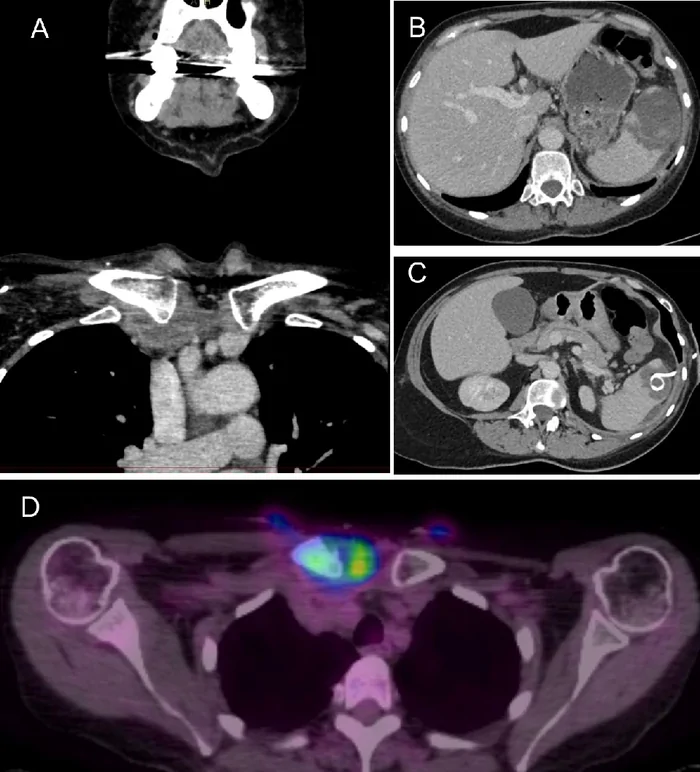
(A) Coronal reformation of a contrast-enhanced computed tomography (CT) scan shows thickening and hyperenhancement of the synovium of the right sternoclavicular joint with associated joint effusion. (B) Axial contrast-enhanced CT image shows a hypodense lesion located along the anterior aspect of the spleen before percutaneous drainage. (C) Axial contrast-enhanced CT image obtained 3 days after percutaneous drainage shows a marked reduction in lesion size. (D) Axial positron emission tomography image shows hypermetabolism in the right sternoclavicular joint and adjacent soft tissues.
